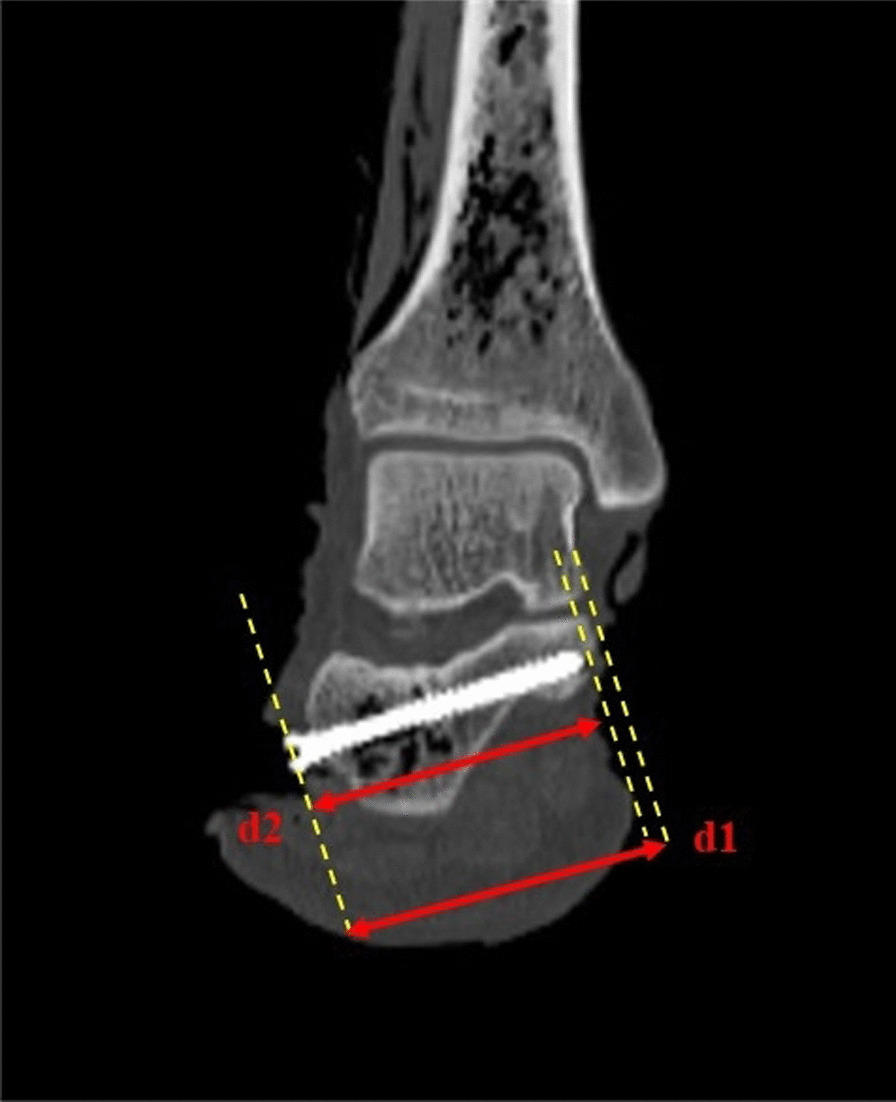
Oblique coronal CT image of ankle joint. (d1) Maximum length through the central axis of the screw; (d2) length of the screw
